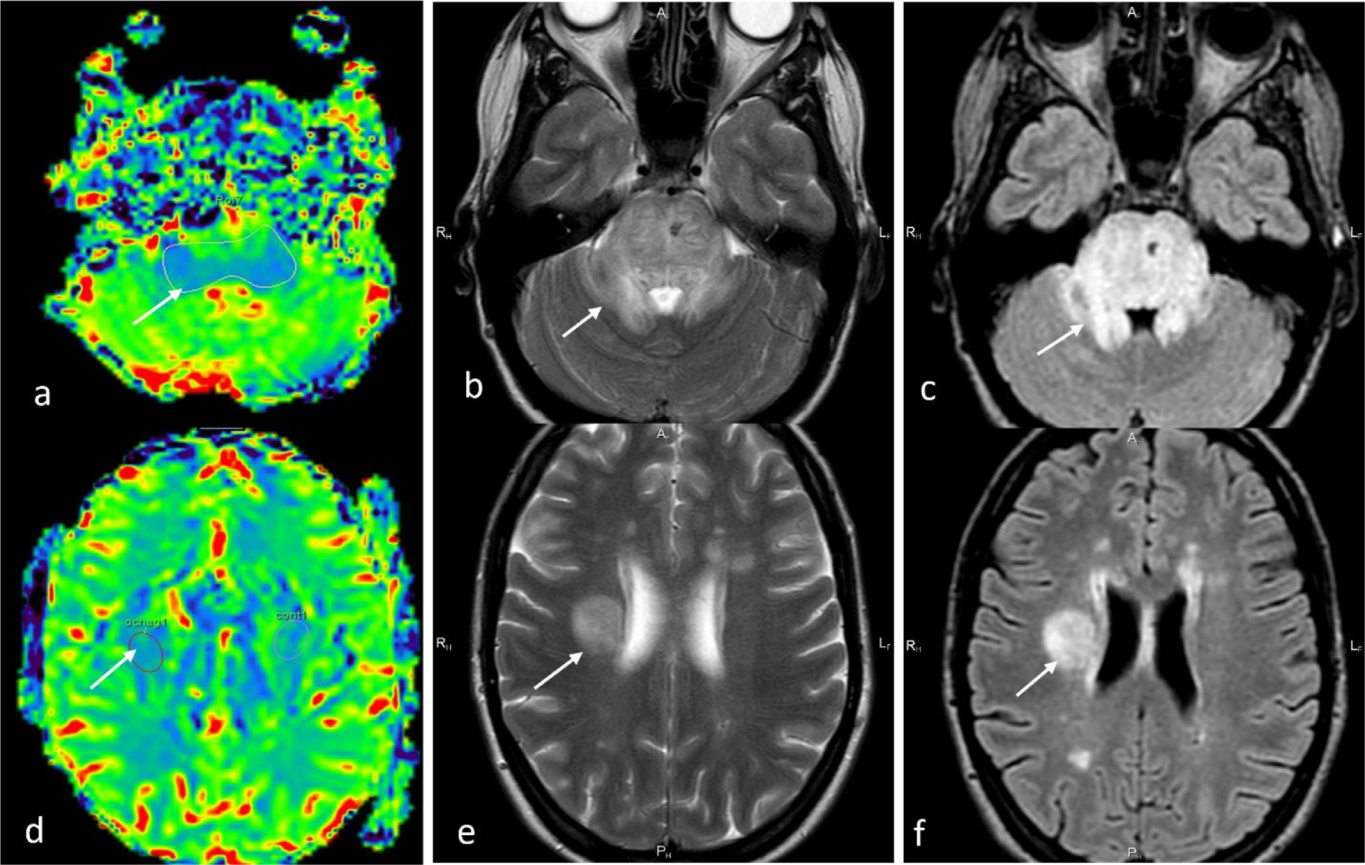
Initial PWI-derived regional CBF maps (a, d) with correlated T2 (b, e) and FLAIR (c, f) images showing decreased regional CBF in the affected pons tissue and in a white matter lesion in the right parietal lobe (CBF by 31% and cerebral blood volume by 64%).
